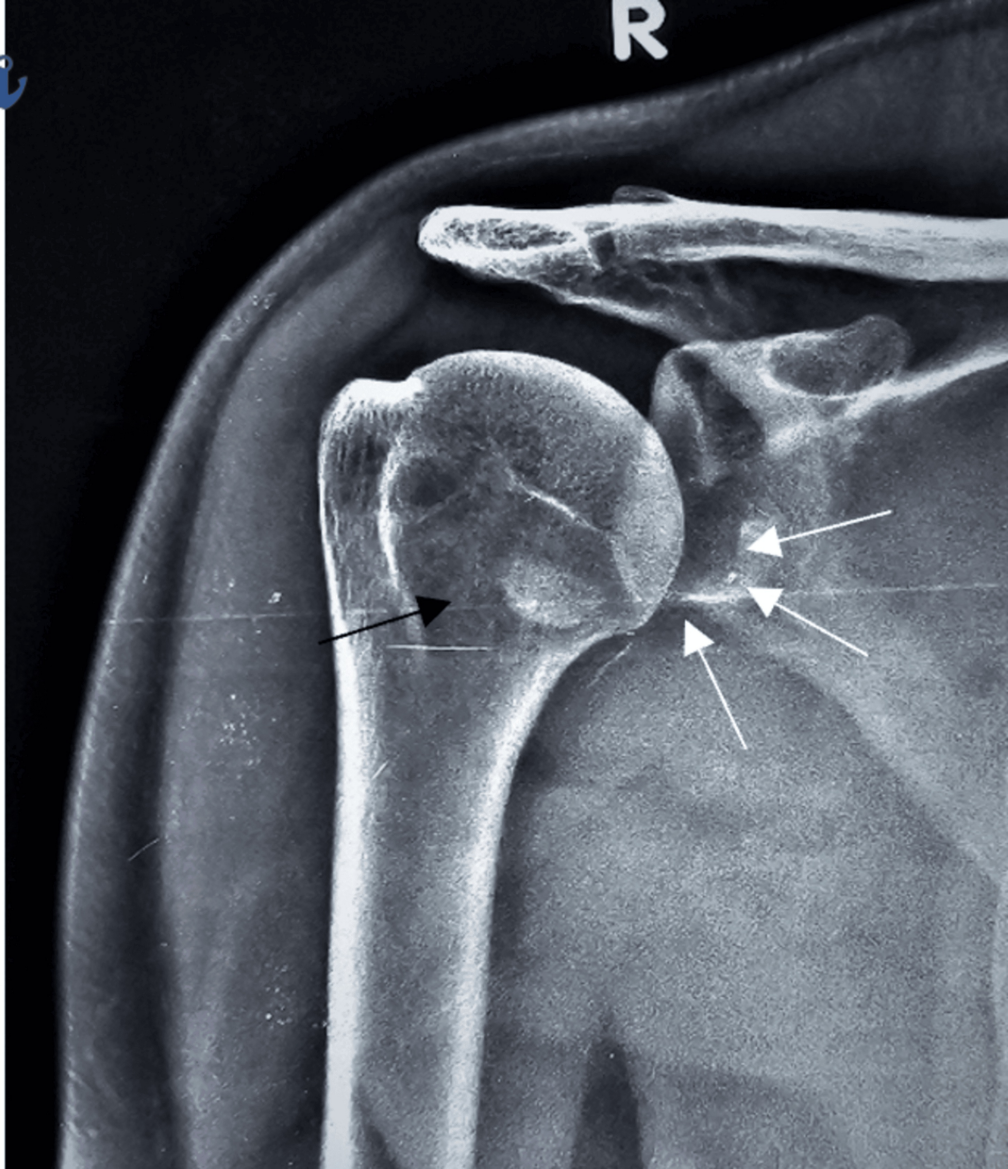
X-ray shows an anterior glenoid rim fracture (indicated by white arrow marks) with a free chondral fragment (shown in the black arrow mark).
